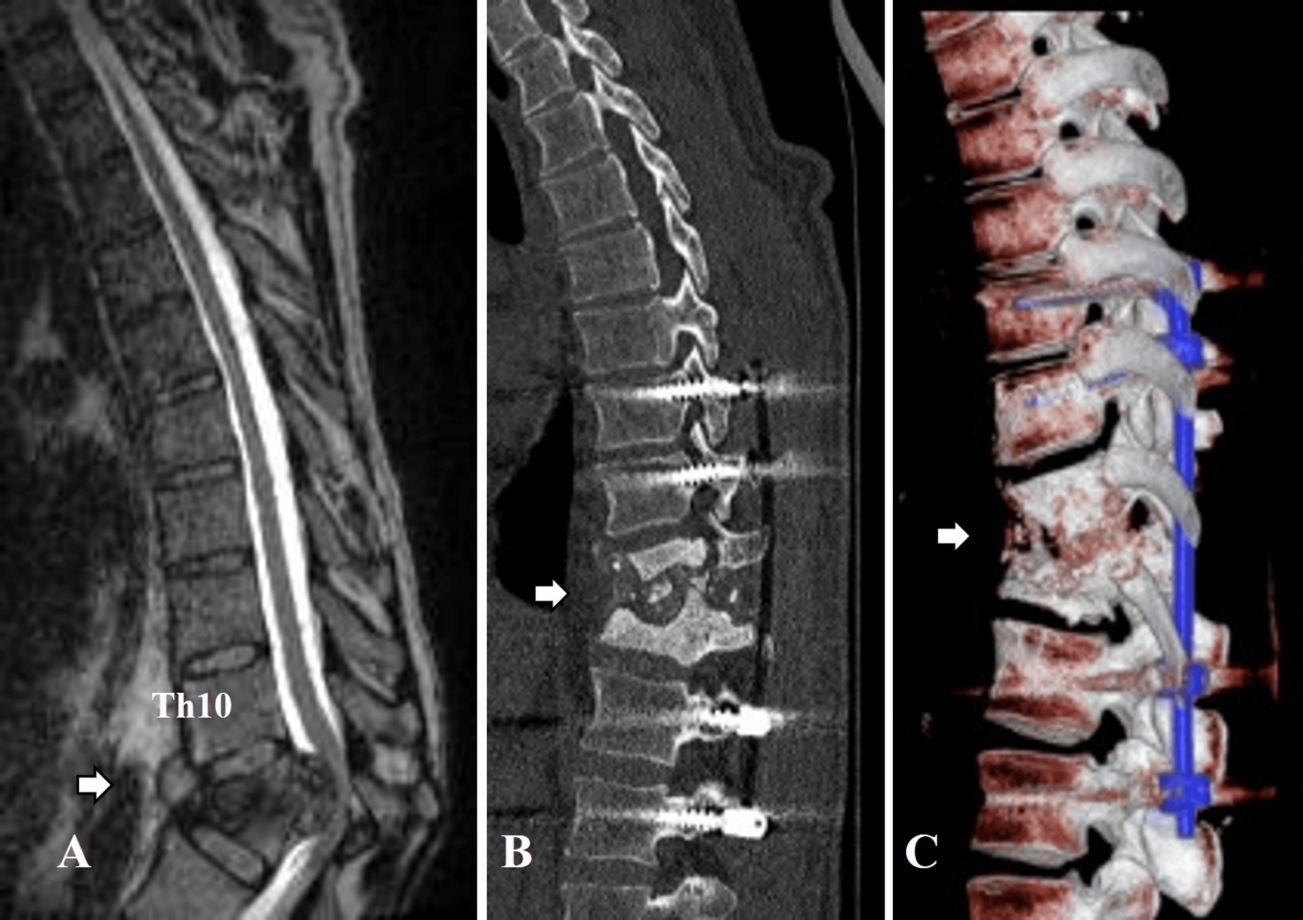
Preoperative and postoperative imaging (Case 2) (A) Preoperative MRI T2 (thick solid arrow); (B) Sagittal postoperative CT reconstruction – shows the achieved alienation of the affected vertebrae and the instrumentation performed (thick solid arrow); (C) Three-dimensional reconstruction (thick solid arrow).
